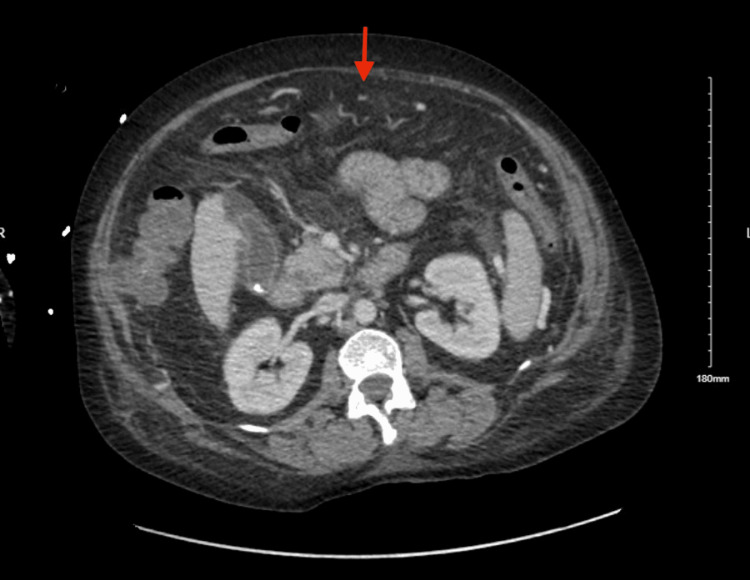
CT enterography There was active extravasation of blood in the right colon.
